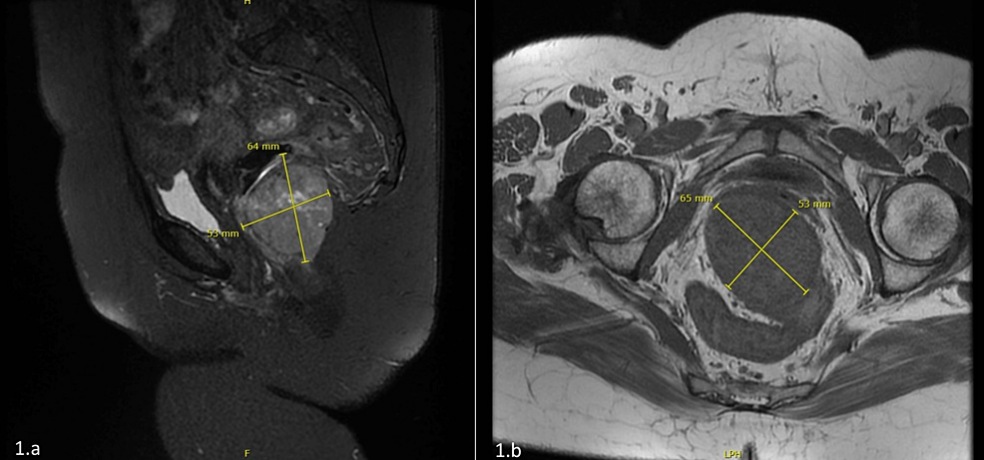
MRI view of the pelvis a: magnetic resonance imaging axial view of the pelvis showing a low rectal mass measuring 6.4 by 5.3 cm. b: magnetic resonance imaging sagittal view of the pelvis
